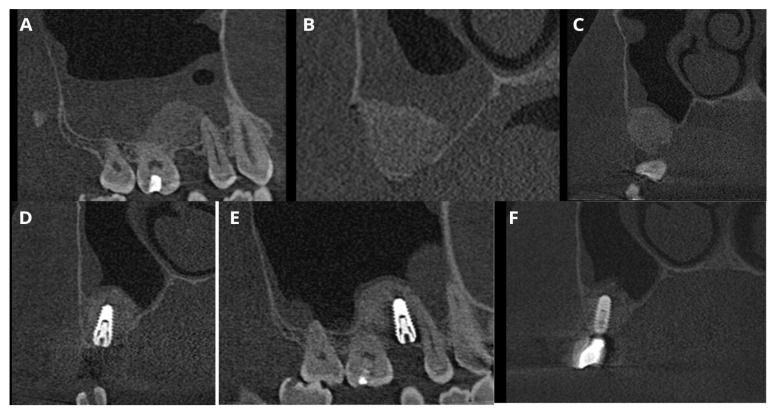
Screenshots of the post-op CBCT scans. Immediately after Croco Eye (A,B), 6 months post-op: before (C) and after implant placement (D,E). The latest follow-up was 5 years 2 months post-op with a loaded implant (F). Throughout the follow-up period, no recurrence of the cyst was detected.
